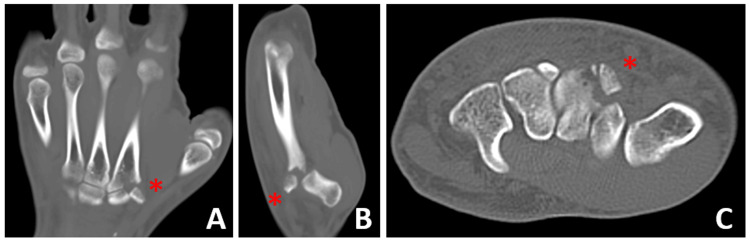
(A) Coronal, (B) sagittal, and (C) axial CT scans showing ECRL avulsion fracture at the base of the second metacarpal bone Red asterisks indicate the avulsed fragment. ECRL, extensor carpi radialis longus
